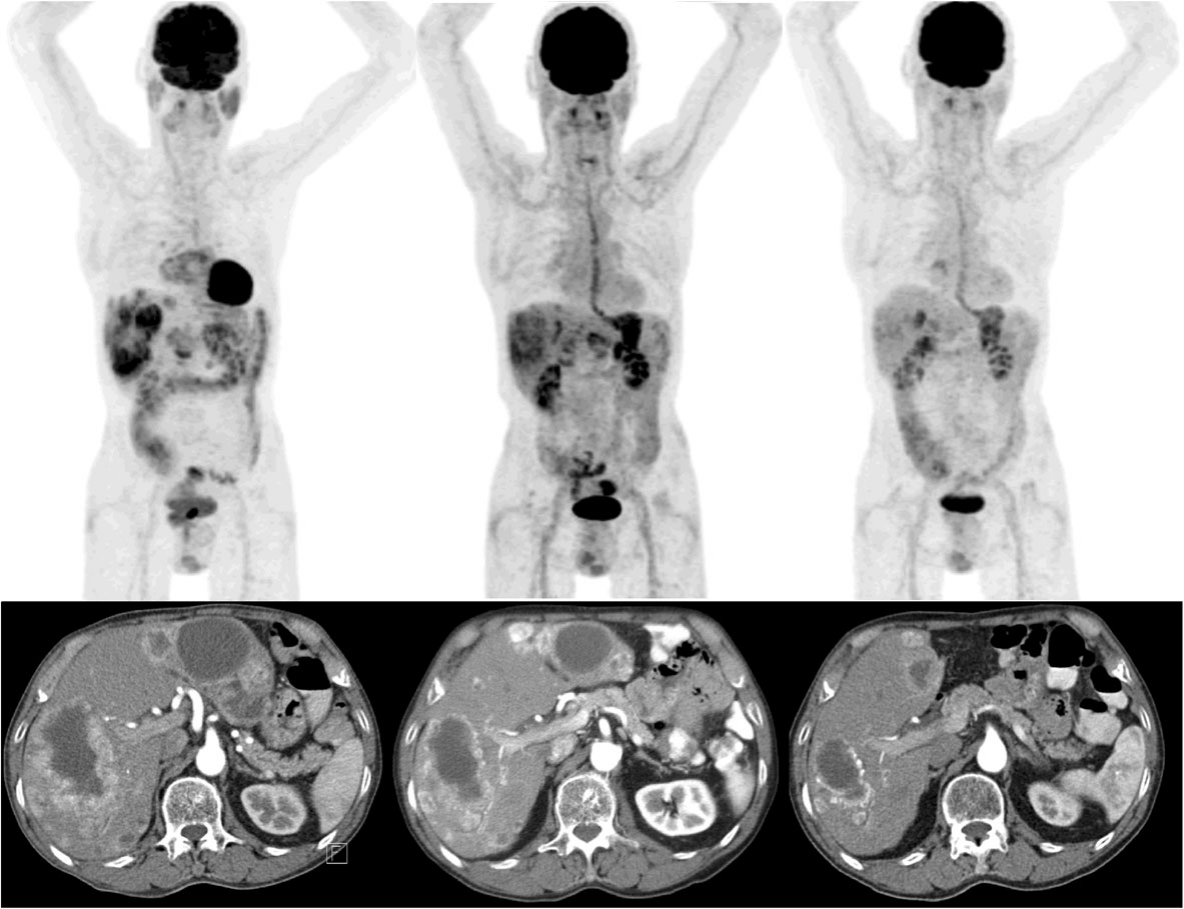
Example of ^166^Ho-radioembolization in NET. A patient with a grade 2 small intestinal NET according to the WHO-criteria, treated in the prior HEPAR 2 trial. On the left, the ^18^FDG-PET and venous phased CT at baseline. In the middle, the imaging studies 3 months after ^166^Ho-RE with partial metabolic ^18^FDG-PET response and some tumour reduction on CT. On the right, follow-up imaging studies 6 months after ^166^Ho-RE with significant partial metabolic response and significant tumour reduction on CT
